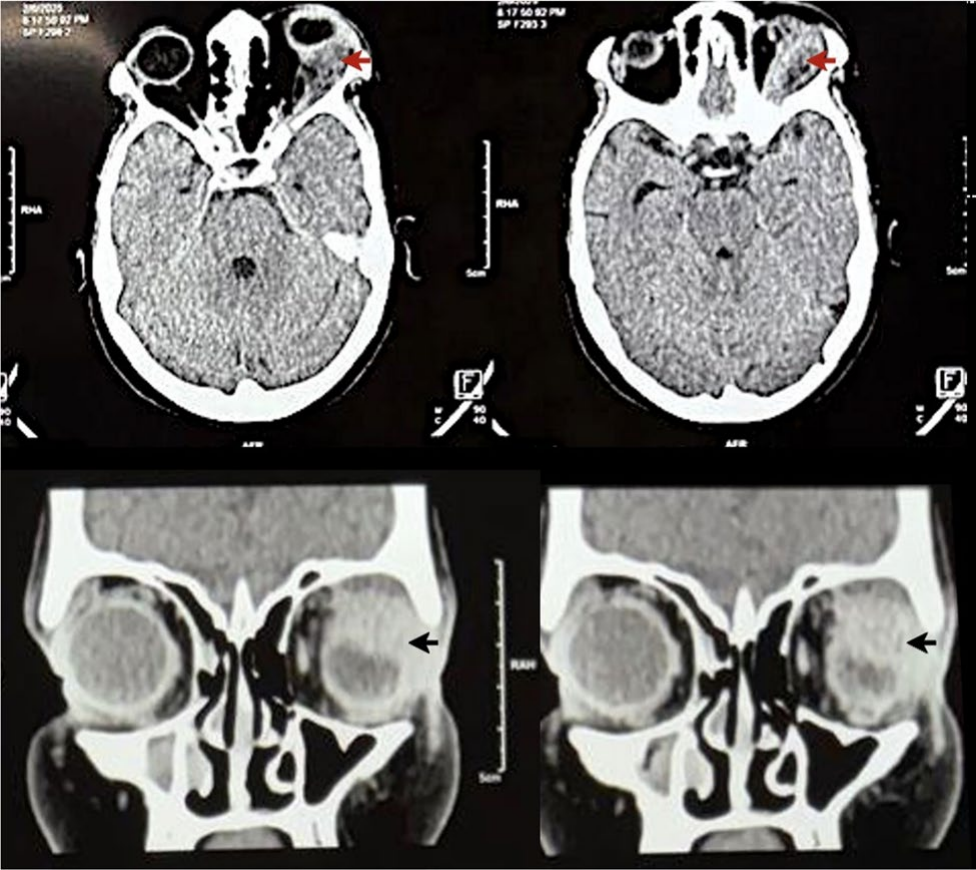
Contrast CT orbit images axial and coronal sections showing ill-defined trans spatial (involving extra and intraconal space) soft tissue density lesion (red and black arrows) measuring – 1.8 × 3.4 cm, epicentered in left lacrimal fossa showing no evident post contrast enhancement with adjacent conal fat stranding causing compression on the posterior segment of left globe and causing proptosis
